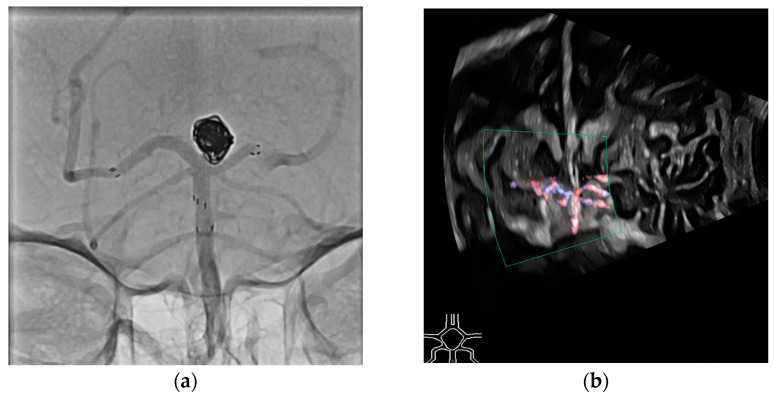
Digital subtraction angiography (a) and duplex ultrasound (b) of the basilar artery treated with a Y-stent. Clear perfusion is visible in the duplex ultrasound, and in this case, the need for follow-up CT angiography to rule out arterial occlusion was avoided. This patient also recovered well and was soon transferred to rehabilitation.
